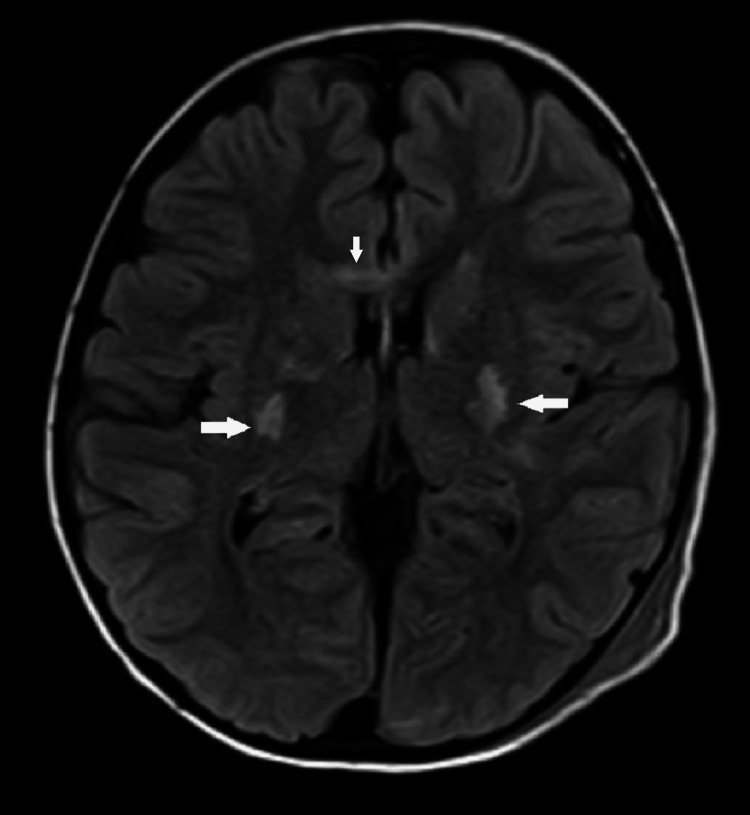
MRI brain axial FLAIR images depicting symmetrical hyperintensities (white arrows) involving bilateral basal ganglia and genu of corpus callosum FLAIR: fluid-attenuated inversion recovery
